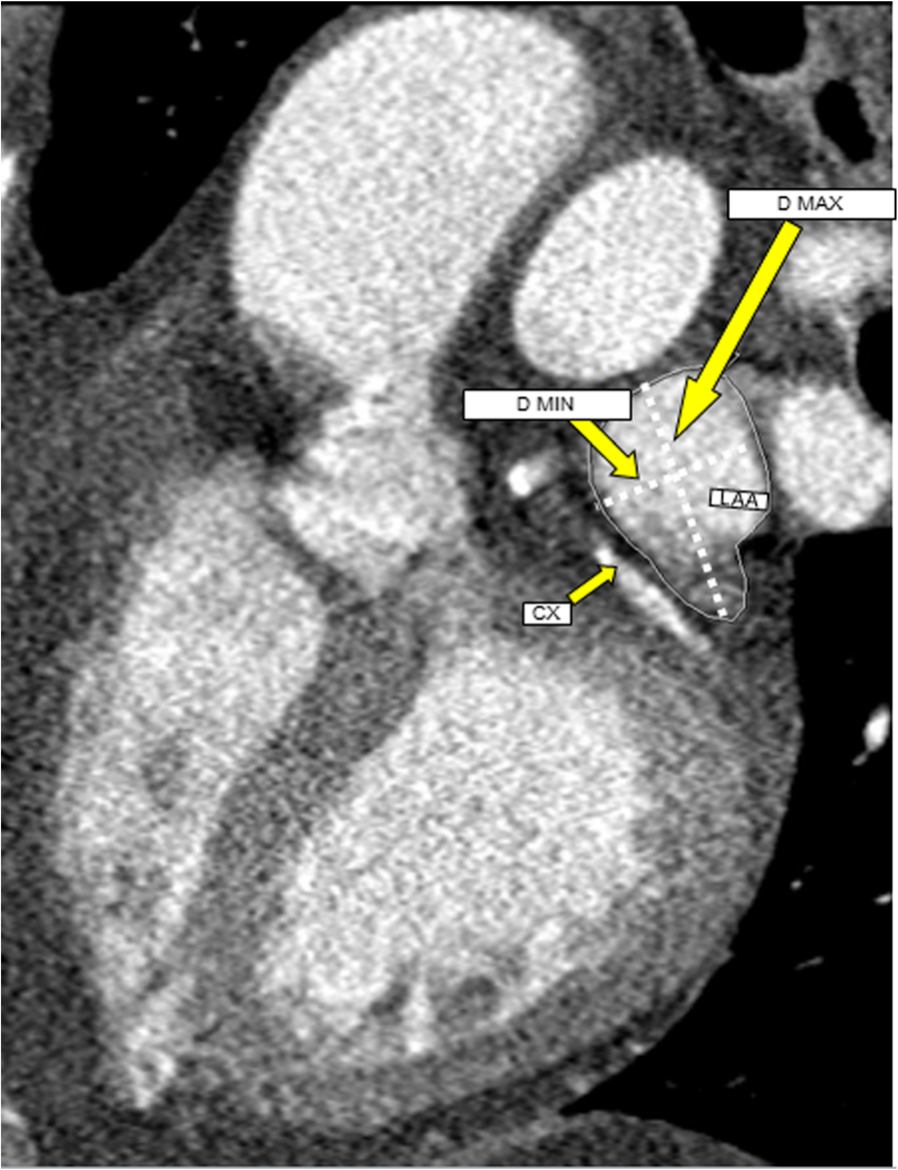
CT images of LAA orifice maximal diameter and area at the level of circumflex (Cx) artery (arrow). (D_max_ = largest diameter, D_min_ = minimal diameter)
